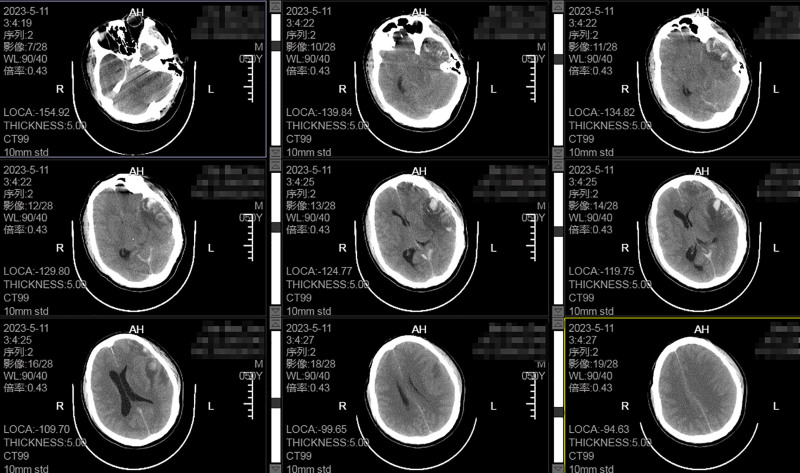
The preoperative CT scan demonstrated compression of the left lateral ventricle, rightward midline shift, obliteration of the suprasellar cistern and perimesencephalic cisterns, and significant cerebral edema, indicating the necessity for surgical intervention. CT = computed tomography.
